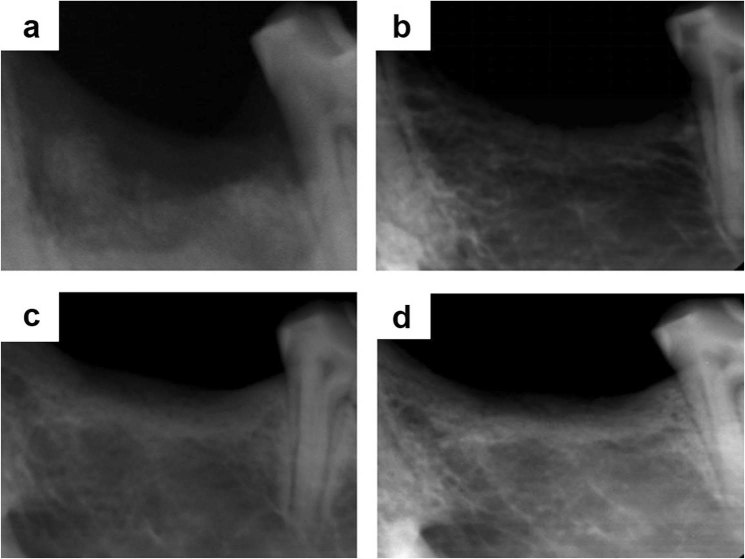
X-radiography showed the different implantation areas after 12w ((a): BC, (b): 3D-BG, (c): 3D-BG + BMP/CS, (d): 3D-BG + rBMSCs + BMP/CS).
